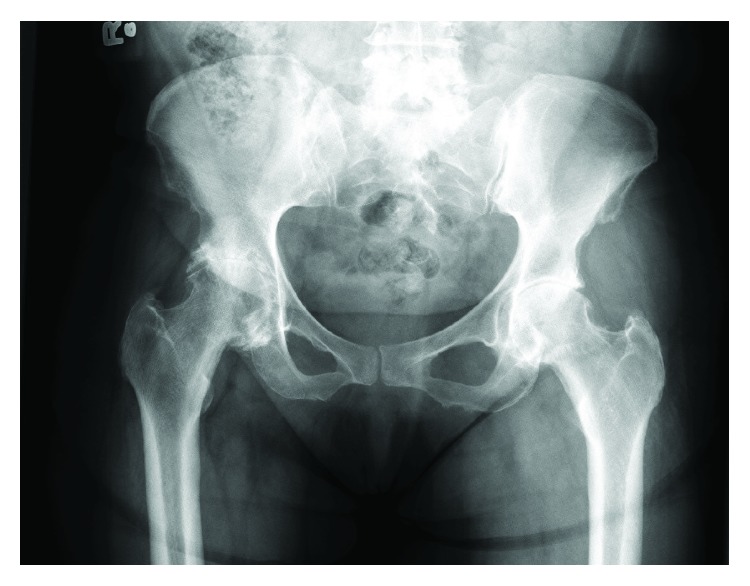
Preoperative AP pelvis radiograph demonstrating advanced degenerative changes of the right hip.
